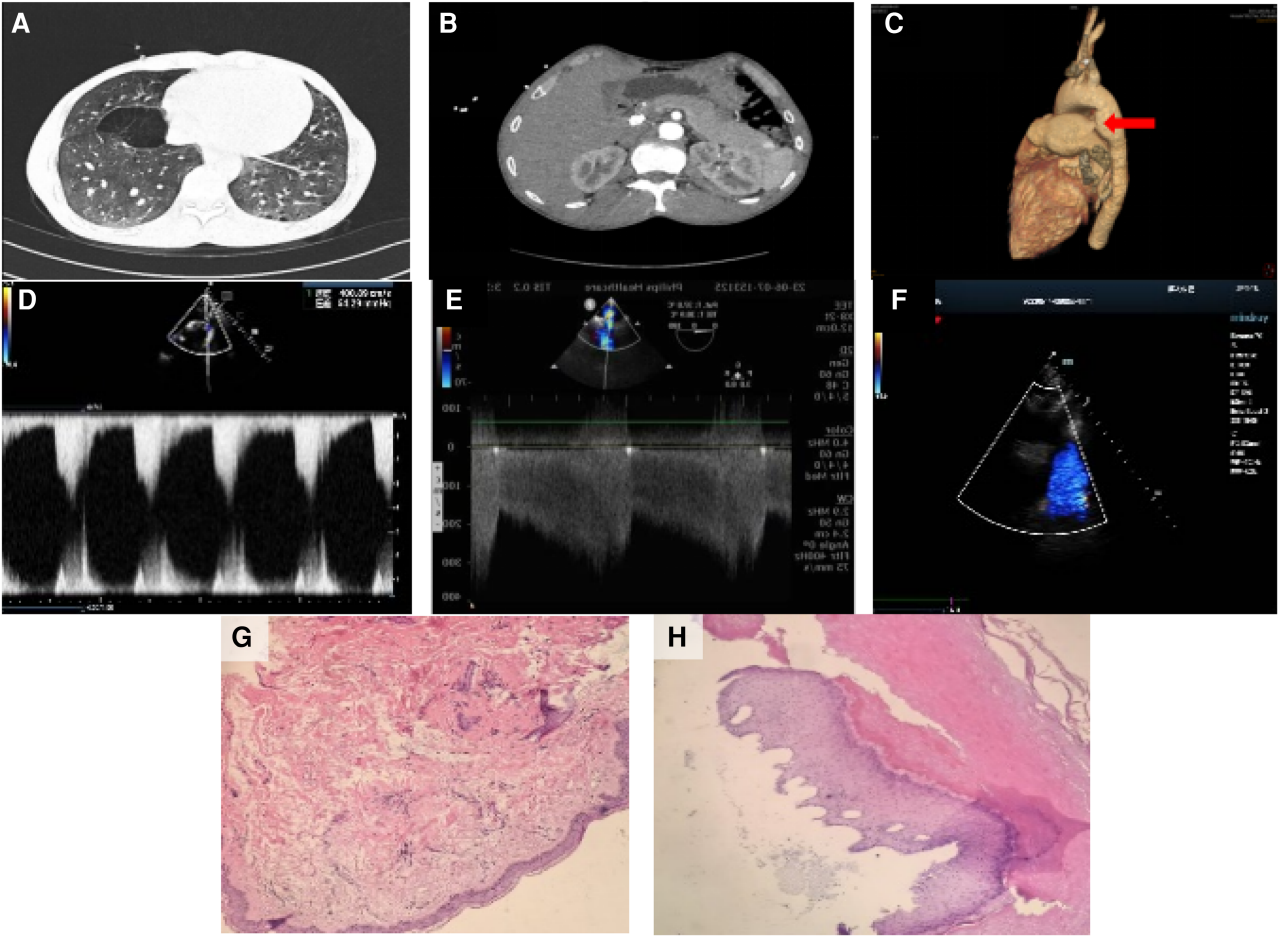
Imaging and pathology results. (A) Several solid nodules in both lungs, with the largest one located in the anterior segment of the right upper lobe, approximately 8 mm × 7 mm in size. Emphysema with pulmonary bullae was also observed in both lungs. Preoperative abdominal plain CT scan. (B) Nodular dense shadow in the liver, nodular dense shadow in the duodenal region, and several nodules in both kidneys, with the largest one having a long diameter of about 64 mm. Preoperative three-dimensional imaging of the thoracic aorta. (C) Fenestration patent ductus arteriosus with pulmonary hypertension. (D) Before the operation, anecho interruption was observed between the origin of the left pulmonary artery and the descending aorta, approximately 13 mm in width (window type), with a continuous left-to-right shunt detected at the level of the large artery. Vmax was approximately 3.2 m/s, and Vmin was approximately 1.7 m/s. (E) During the operation, the occluder-like echo was detected between the origin of the left pulmonary artery and the descending aorta. The position was fixed, the traction test was negative, and no significant blood flow acceleration was detected at the origin of the descending aorta and the origin of the left pulmonary artery. (F) After the operation, the thickness of the interventricular septum and left ventricular posterior wall was normal. The overall motion amplitude of the left ventricular wall was slightly decreased, the diameter of the ascending aorta was widened, and the occluder-like hyperecho was detected between the origin of the left pulmonary artery and the descending aorta. (G) Pathological examination of the skin on the left waist showed hyperkeratosis of the epidermis and a few lymphocytes infiltration around the small vessels of the dermis. (H) Pathological examination of the skin on the left foot also showed hyperkeratosis of the epidermis and a few lymphocytes infiltration around the small vessels of the dermis.
